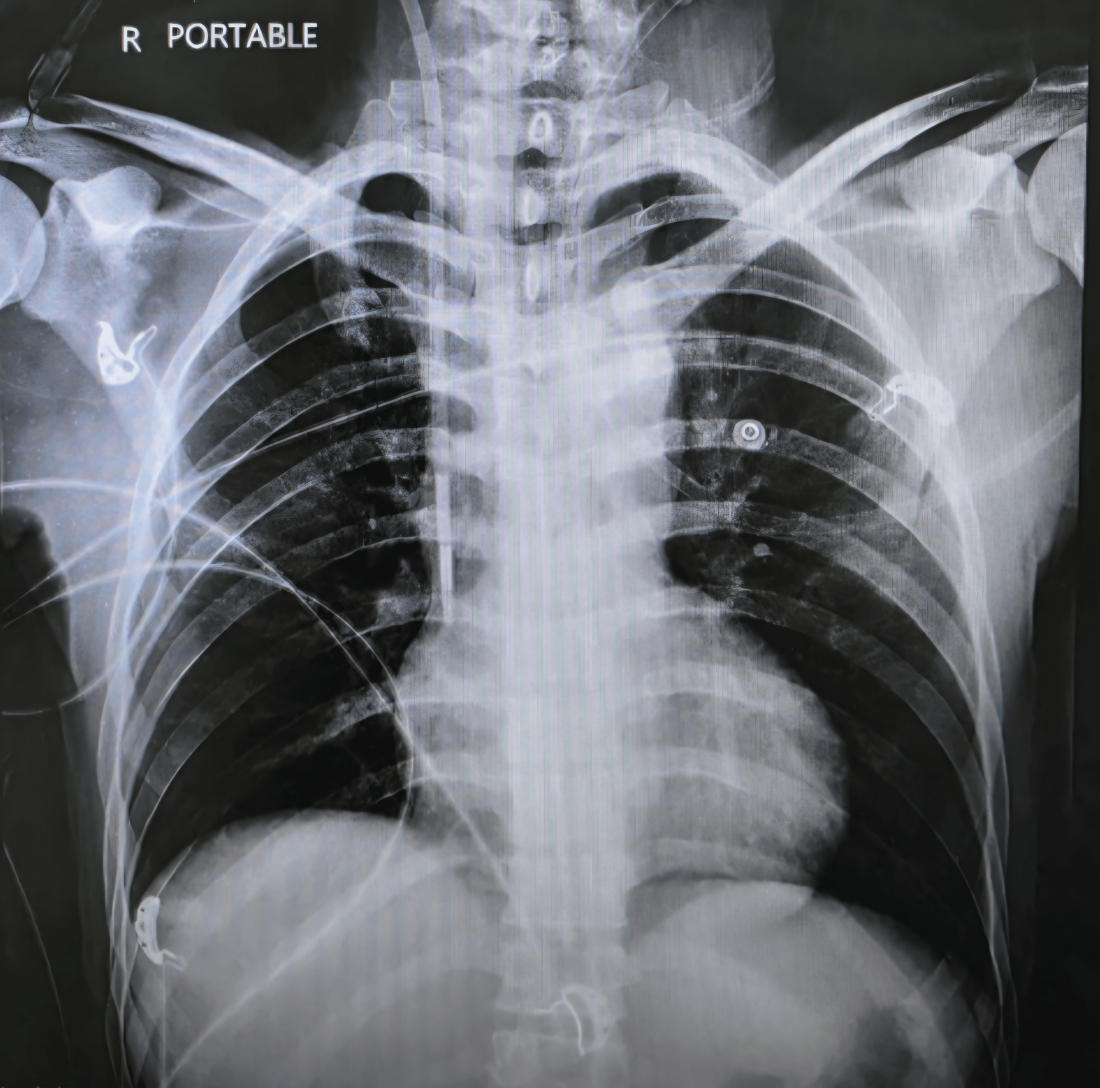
Follow-up chest radiograph on day nine showing resolution of pleural effusion and pulmonary edema
